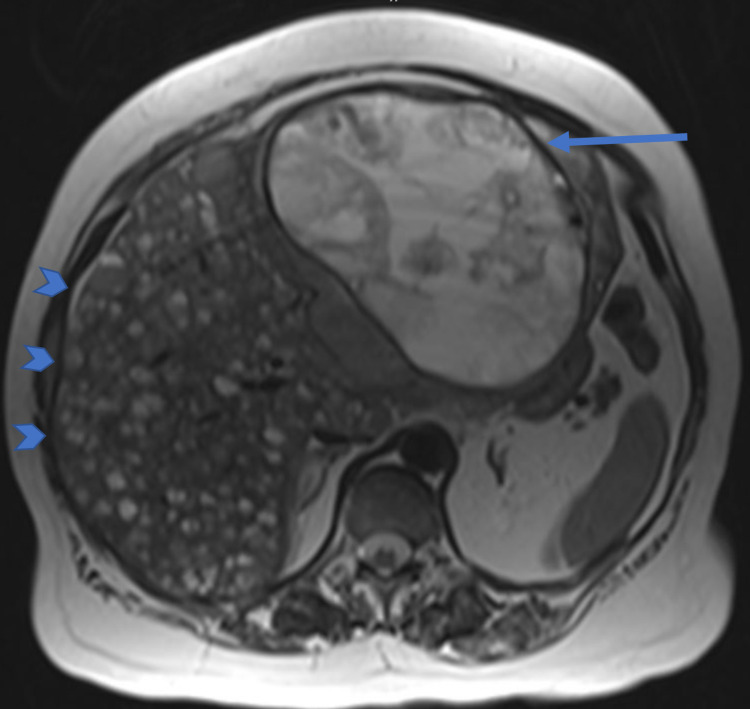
MRI of the abdomen showing an intrinsic T1 hyperintense lesion consistent with a large 14.9 x 14.0 cm complex necrotic mass in the left hepatic lobe (blue arrow), with innumerable small metastatic lesions throughout the liver parenchyma (blue arrowheads).
